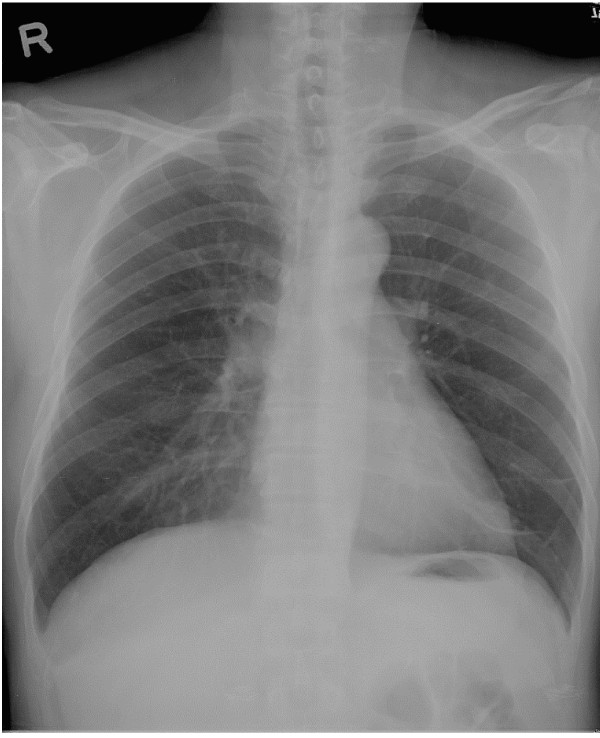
Chest X-ray showing a mass shadow over the left lower lung field (arrow).
